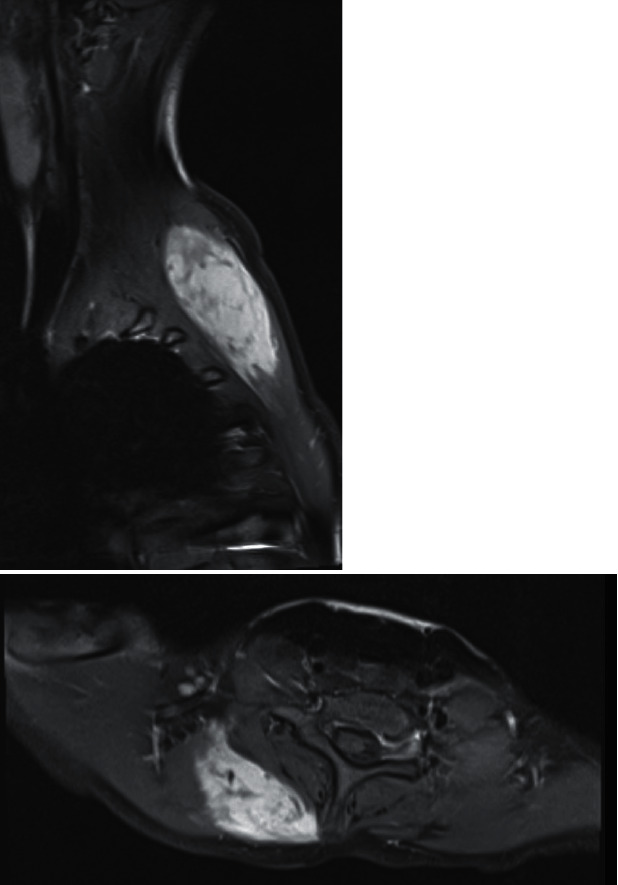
Right parasagittal and axial T1-weighted MRI sections demonstrating gadolinium contrast-enhancing right paraspinal mass consistent with a desmoid tumour.
